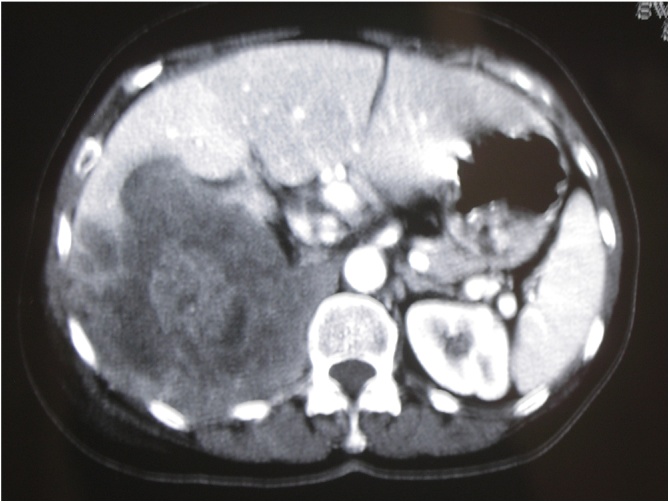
CT abdomen revealing a mass with a transverse diameter of 12 cm in the retroperitoneal region.
